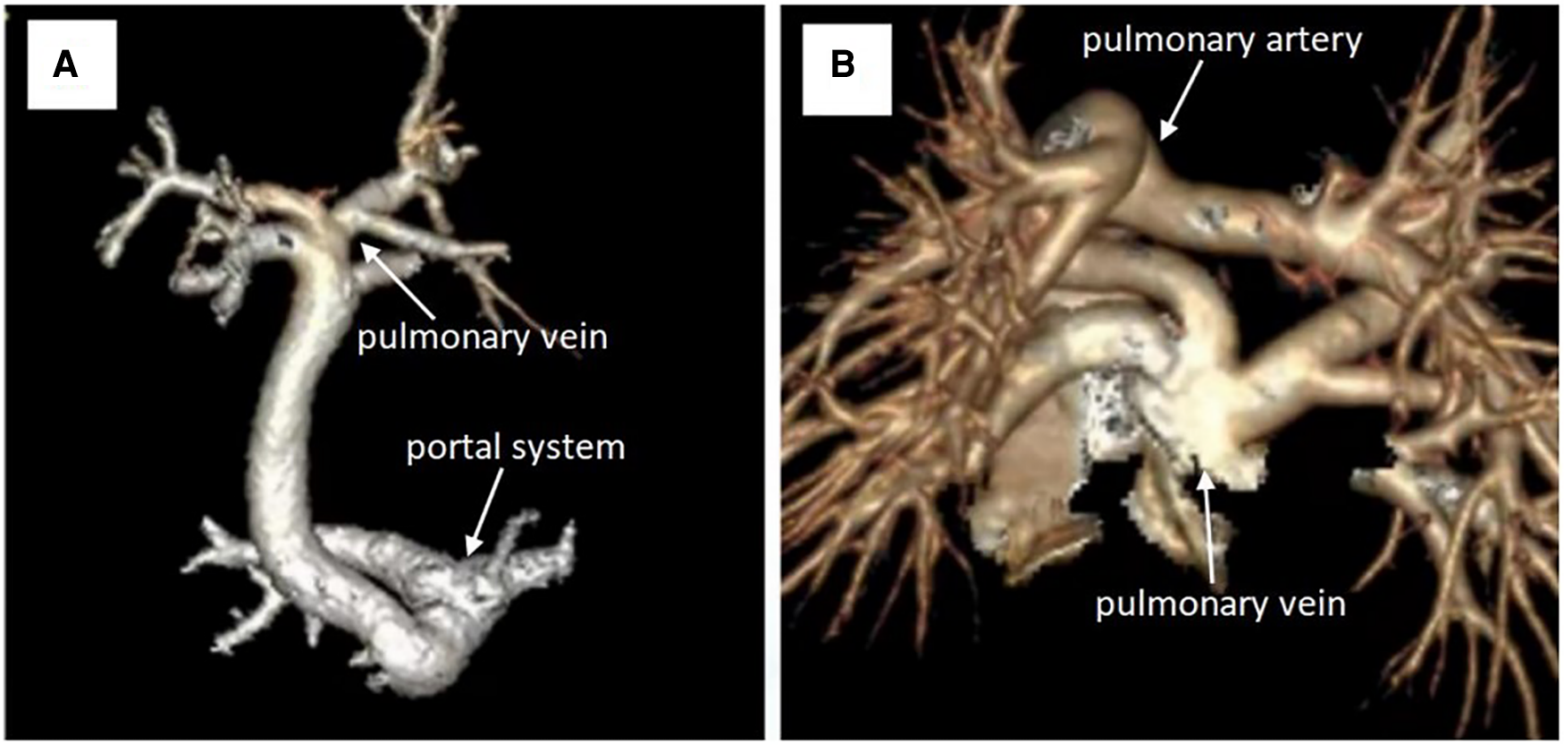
(A) CT angiography reconstruction for the preoperative pulmonary vein. (B) CT angiography reconstruction for the postoperative pulmonary vein .
